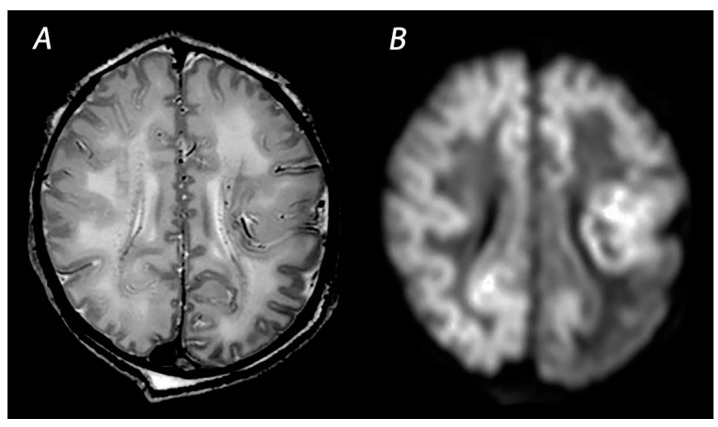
MRI was performed on day 3 in an infant born with Apgar scores of 9/10 who developed intractable seizures within the first hours after birth. T2 weighted imaging shows the decreased distinction of the gray and white matter at the cortex (A). DWI shows extensive diffusion restriction with sparing of the anterior periventricular white matter and an asymmetrical distribution of white matter injury in the parieto-occipital lobes (B). The infant died on day 5 after redirection of care and was subsequently diagnosed with Molybdenum cofactor deficiency.
